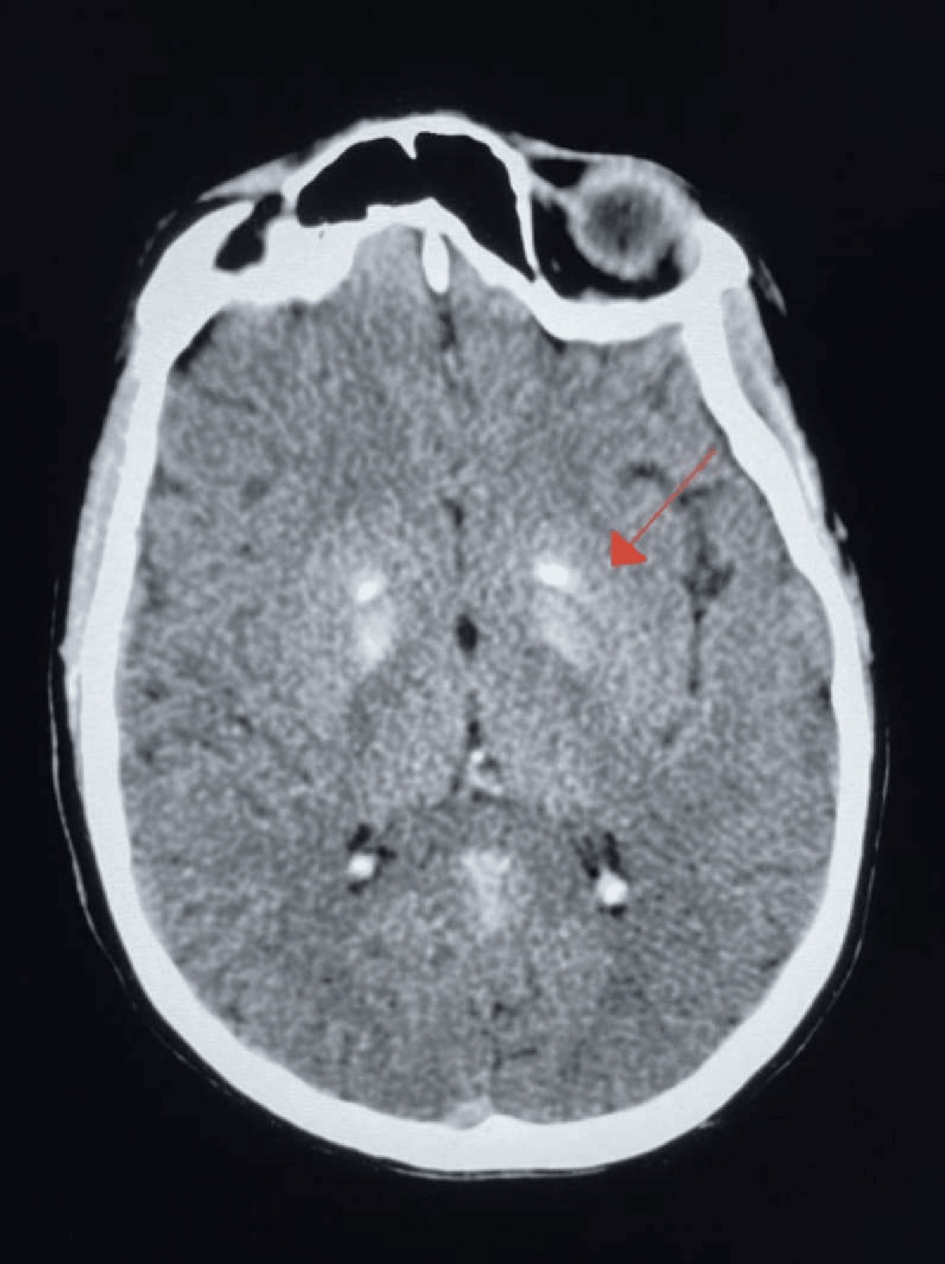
CT imaging of the head in the axial plane showing bilateral basal ganglia calcification in a tram-track-like pattern (red arrow).
